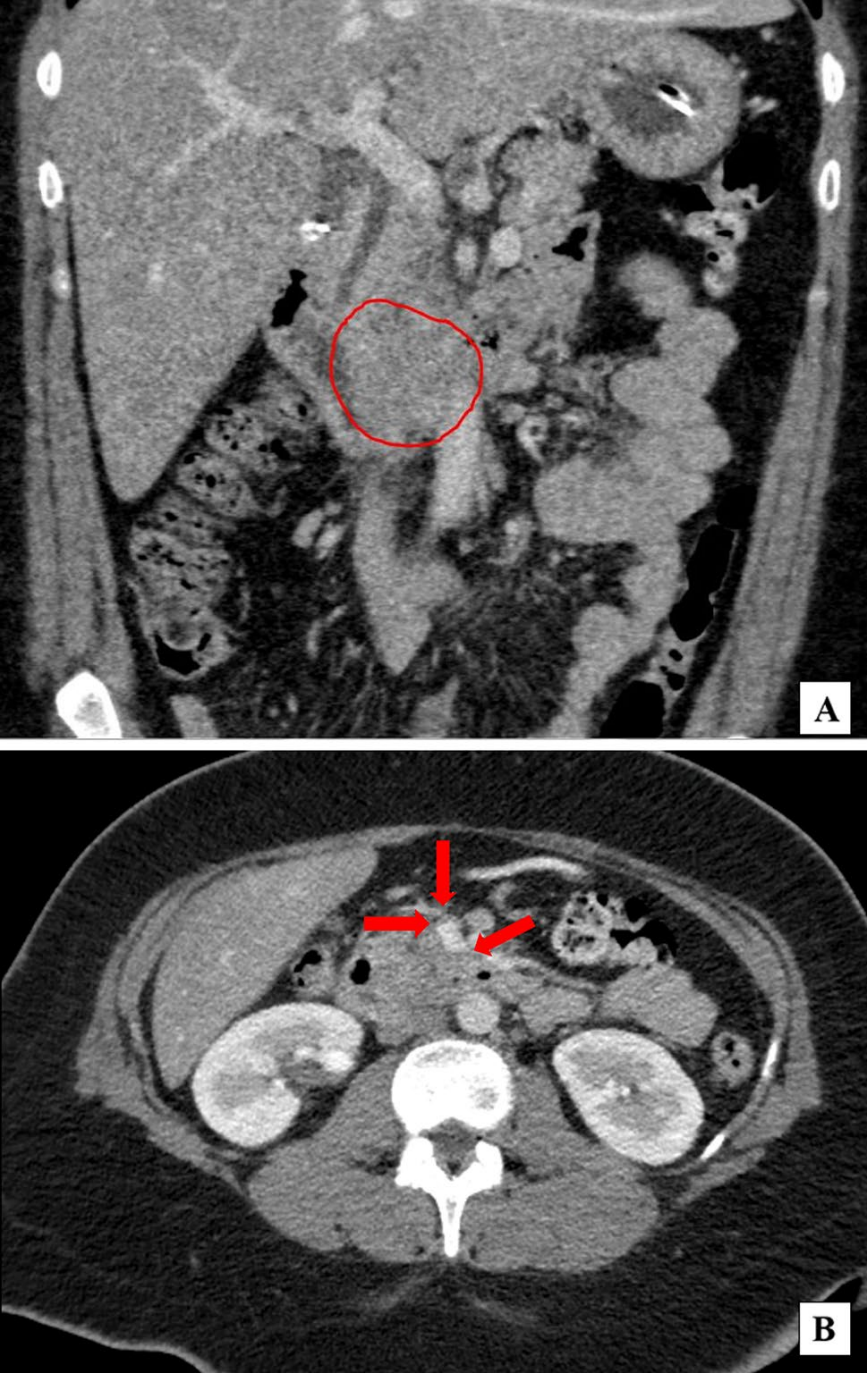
Coronal and axial abdominal CT images with intravenous contrast demonstrating duodenal mass and adjacent vessels. A Red circle encompasses 4.5 cm × 2.6 cm mass in the proximal third portion of the duodenum at the inferior duodenal flexure causing duodenal narrowing and biliary obstruction. B Red arrows point to locally advanced tumor, which is abutting the superior mesenteric vessels. The vessels remain patent
